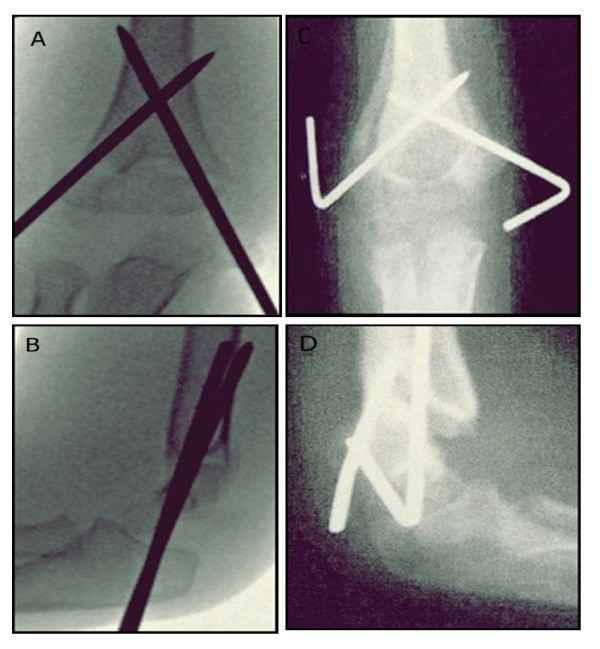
A, Immediate postoperative Anteroposterior radiograph of crossed medial-lateral entry pinning showing acceptable reduction. B, Immediate postoperative lateral radiograph of crossed medial-lateral entry pinning showing acceptable reduction. C, Follow-up Anteroposterior radiograph showing loss of reduction in crossed medial-lateral entry pinning. D, Follow-up lateral radiograph showing loss of reduction in crossed medial-lateral entry pinning.
